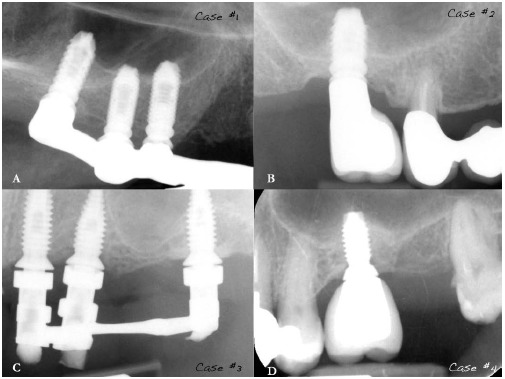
Periapical x-rays of cases #. 1 (a), #. 2 (b), #. 3 (c) and #. 4 (d) showing no pathological signs respectively on implant #. 1.6 after 6 years from the grafting procedure, on implant #. 1.6 after 3 years, on implant #. 2.6 after 4 years, and on implant #. 2.6 after 3 years. The gap visible between implants and abutments is due to the presence of a radiolucent shock adsorber considered by the used implant system.
